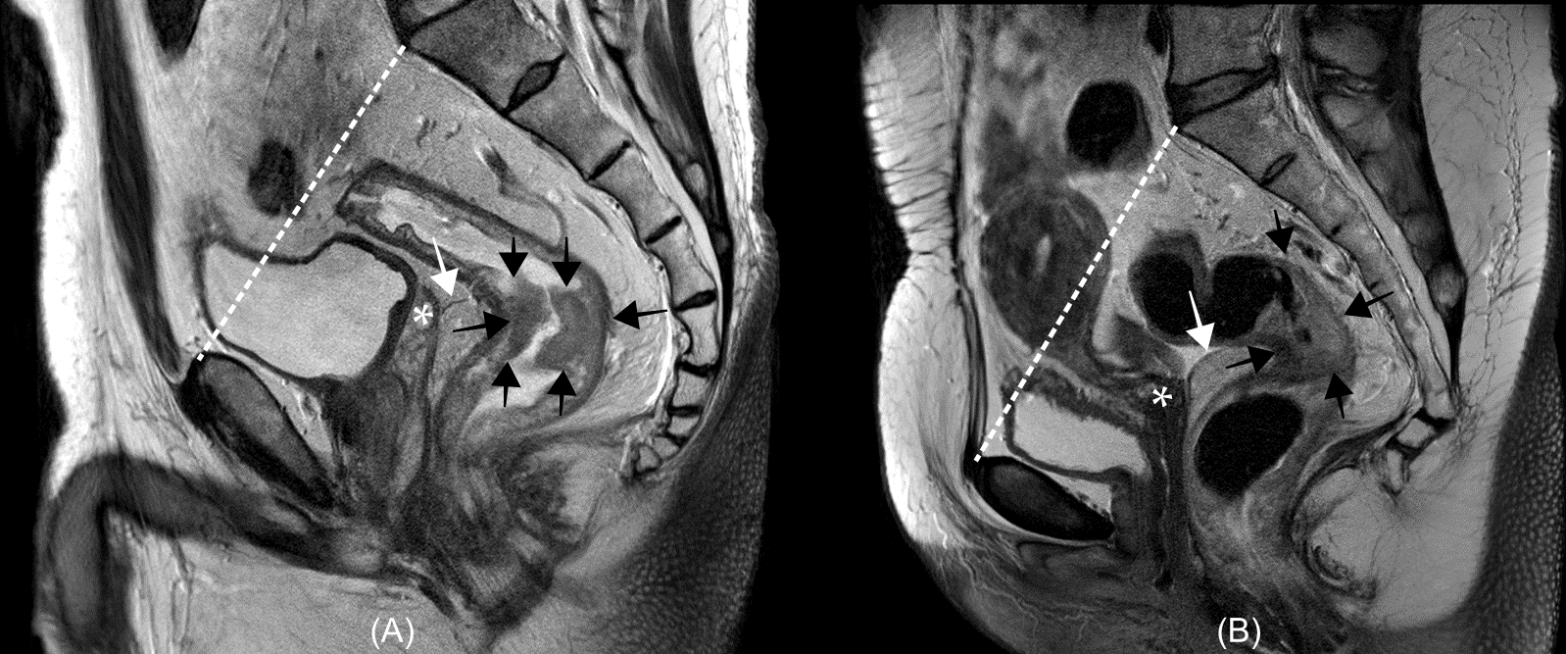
Examples of rectum anatomy on magnetic resonance image from a man (A) and a woman (B) with rectal cancer. In the National Comprehensive Cancer Network (NCCN) guidelines, the rectum is defined as the portion of the bowel located below an imaginary line drawn from the sacral promontory to the top of the pubic symphysis (white dashed lines). The anterior peritoneal reflections (white arrows) is located around the upper border of the seminal vesicle [asterisk in (A)] in the man or uterocervical angle [asterisk in (B)] in the woman. In the NCCN guidelines, the rectum is divided according to the relative location from the anterior peritoneal reflection: upper rectum, above the anterior peritoneal reflection; mid-rectum, at the anterior peritoneal reflection; and lower-rectum, below the anterior peritoneal reflection. Rectal cancers (black arrows) locate below (A) and across (B) the anterior peritoneal reflection.
